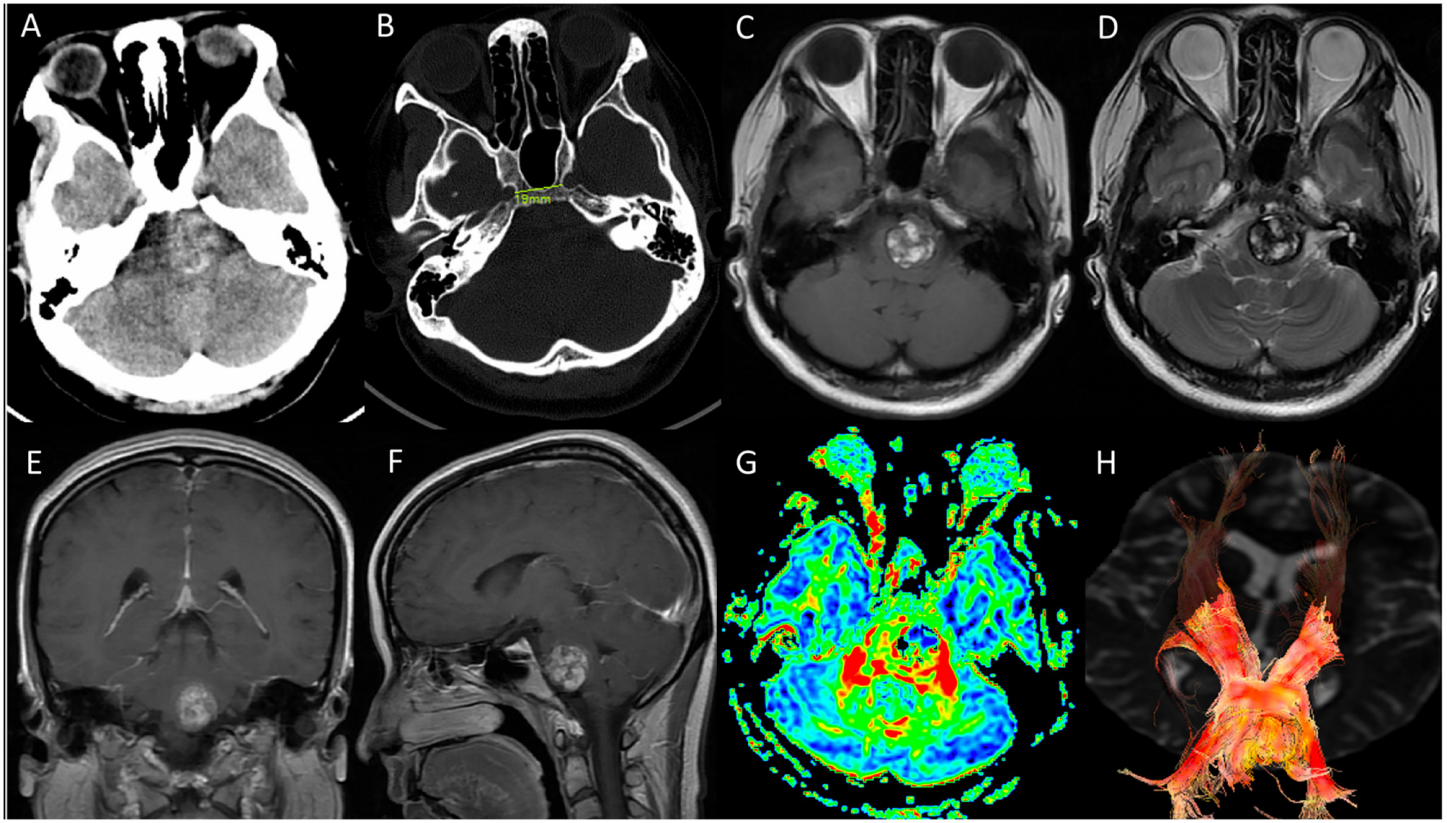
Preoperative imaging. (A,B) CT scan revealed a hemorrhagic occupying lesion in the left pons with acute blood products and indicated a distance of 19 mm between para clival carotid in bone window. The development and the degree of pneumatization of sphenoid sinus was suitable for endoscopic endonasal surgery; (C–F) Axial T1-weighted, T2-weighted, coronal and sagittal T1 postcontrast magnetic resonance image demonstrated a 2.3*2 cm ventral pontine CM with hemosiderin surrounded, and no significant enhancement of the CM was observed; (G,H) diffusion tensor imaging (DTI) showed the corticospinal tract was displaced laterally by the CM.
